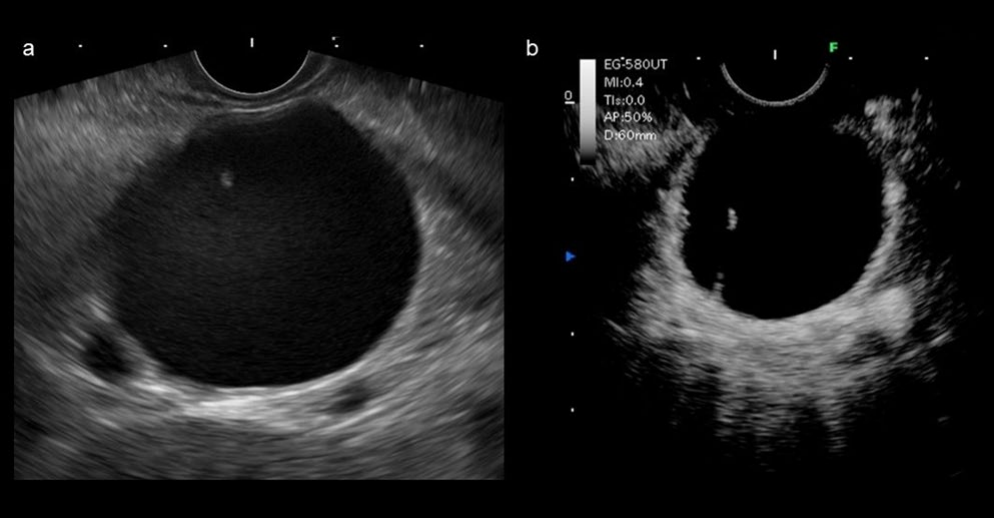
Endoscopic ultrasonography. a Endoscopic ultrasonography (EUS) showed a 37 × 30 mm round cyst in the pancreatic tail. A septate-like structure was observed within the cyst, and the cyst was filled with sludge echoes. b Contrast-enhanced EUS with perflubutane showed early contrast of the cyst wall
